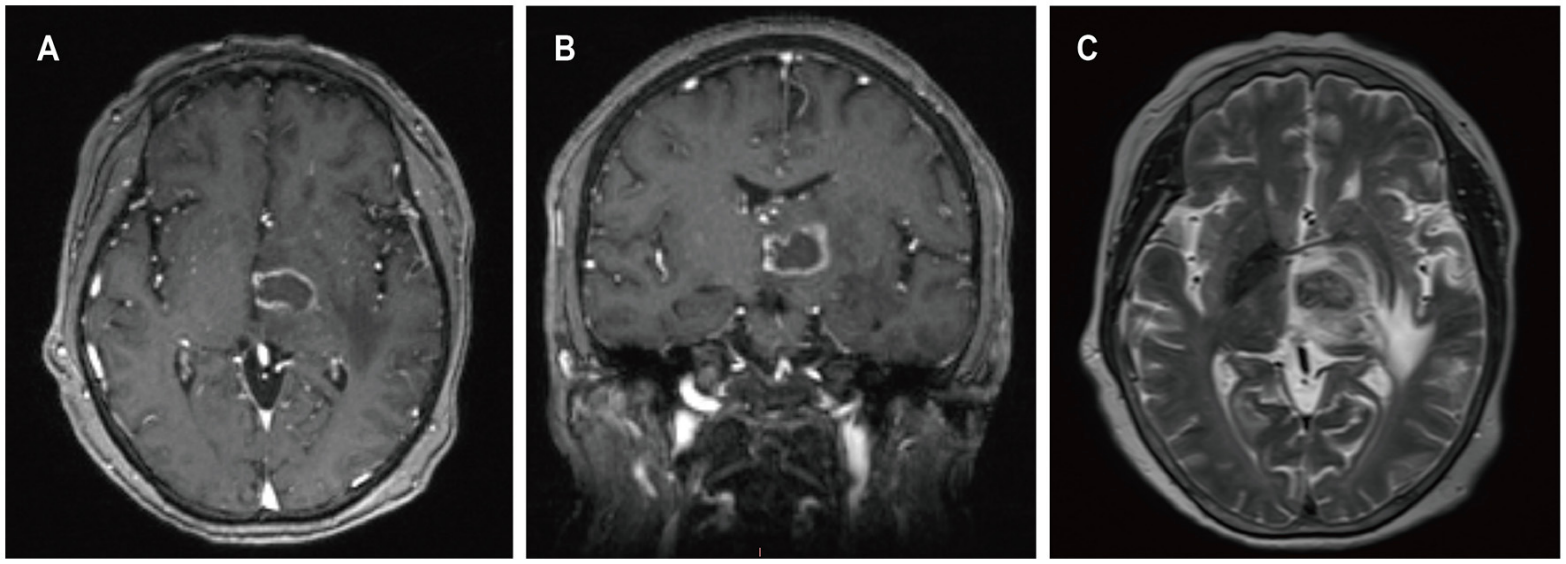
MRI findings of patient 3. Preoperative enhanced MRI prior to brain biopsy showed ring-enhancing lesions affecting the left cerebral peduncle, thalamus, and basal ganglia on T1-weighted images (A, B), with surrounding edema around the left cerebral lesion on T2-weighted images (C).
